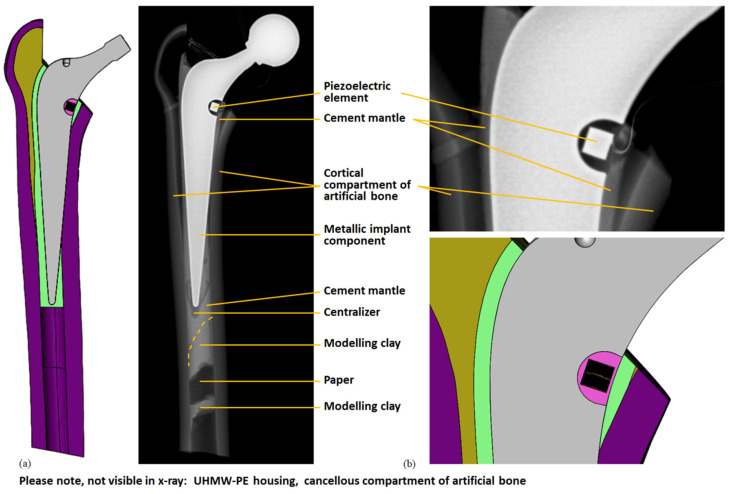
Comparison of virtual and realised implantation. Cross-sectional view of the CAD model (cf. Figure 1) and X-ray image: (a) energy harvesting system with artificial bone; (b) detail of the energy harvesting system. The cables from the piezoelectric element and strain gauge are also visible. The modelling clay and paper were used to prevent the cement from pouring too far into the medullary cavity and the centraliser assured a central position of the distal implant tip; these parts were not contained in the CAD model.
